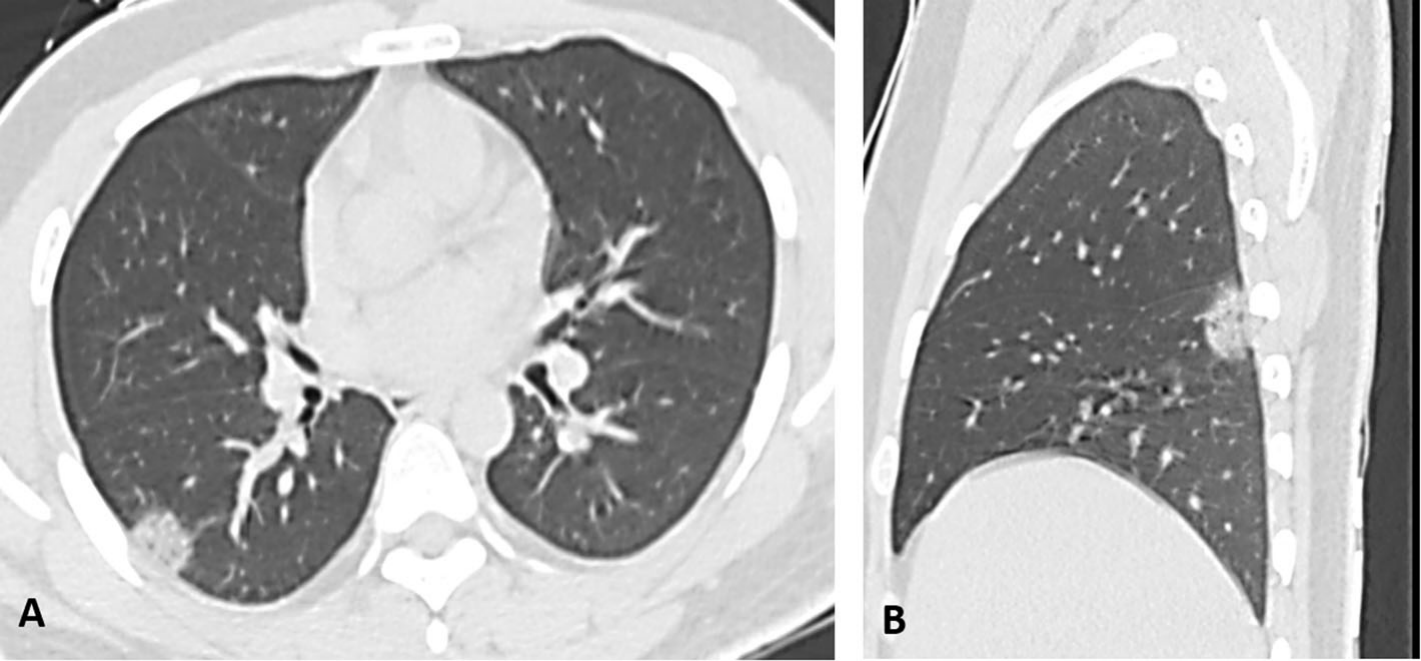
Non-contrast CT of a 36-year-old male with fever, sore throat, and body ache, rt RT-PCR +VE (A and B): A, axial and B, sagittal non-contrast CT scan in lung window show peripheral single ground glass opacity in the apical segment of the right lower lobe
